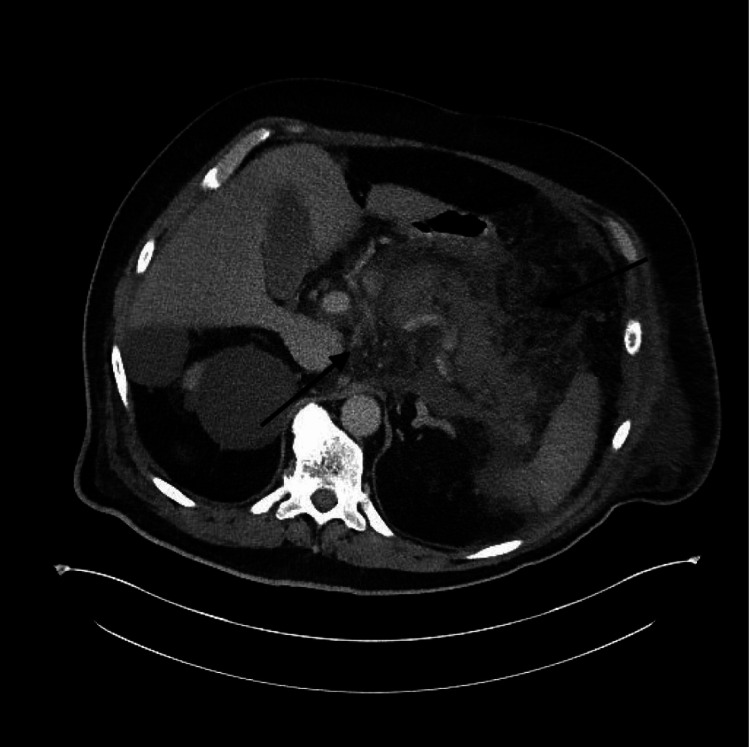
CT abdomen and pelvis shows necrosis in the tail and body of the pancreas
